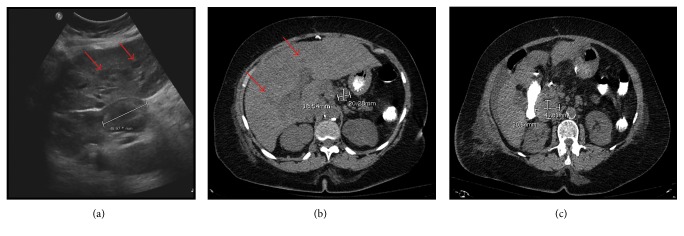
(a) Ultrasound of the liver showing 5 cm enlarged perihepatic lymph node with multiple hypoechoic nodules in the liver (red arrows); (b, c) CT scan showing multiple hypodense nodules in the liver parenchyma (red arrows) with marked enlarged perihepatic lymph nodes.
